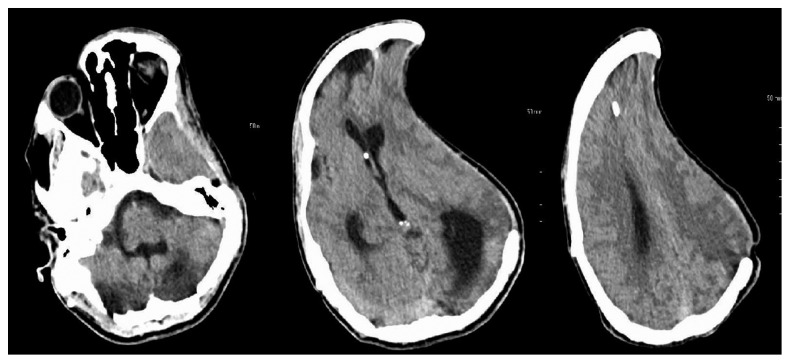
Head CT revealing left-sided prior craniectomy with improved midline shift as compared to Figure 1.
